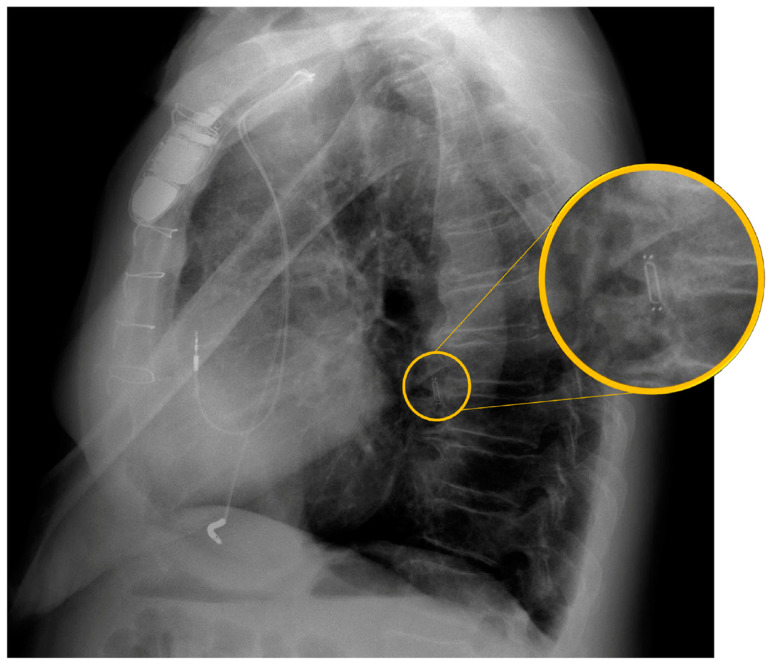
Lateral view X-ray of a patient with a dual chamber implantable cardioverter defibrillator (ICD), showing an implanted CardioMEMS device within a branch of the pulmonary artery. The CardioMEMS device is highlighted in orange, with a zoomed-in view for clarity.
